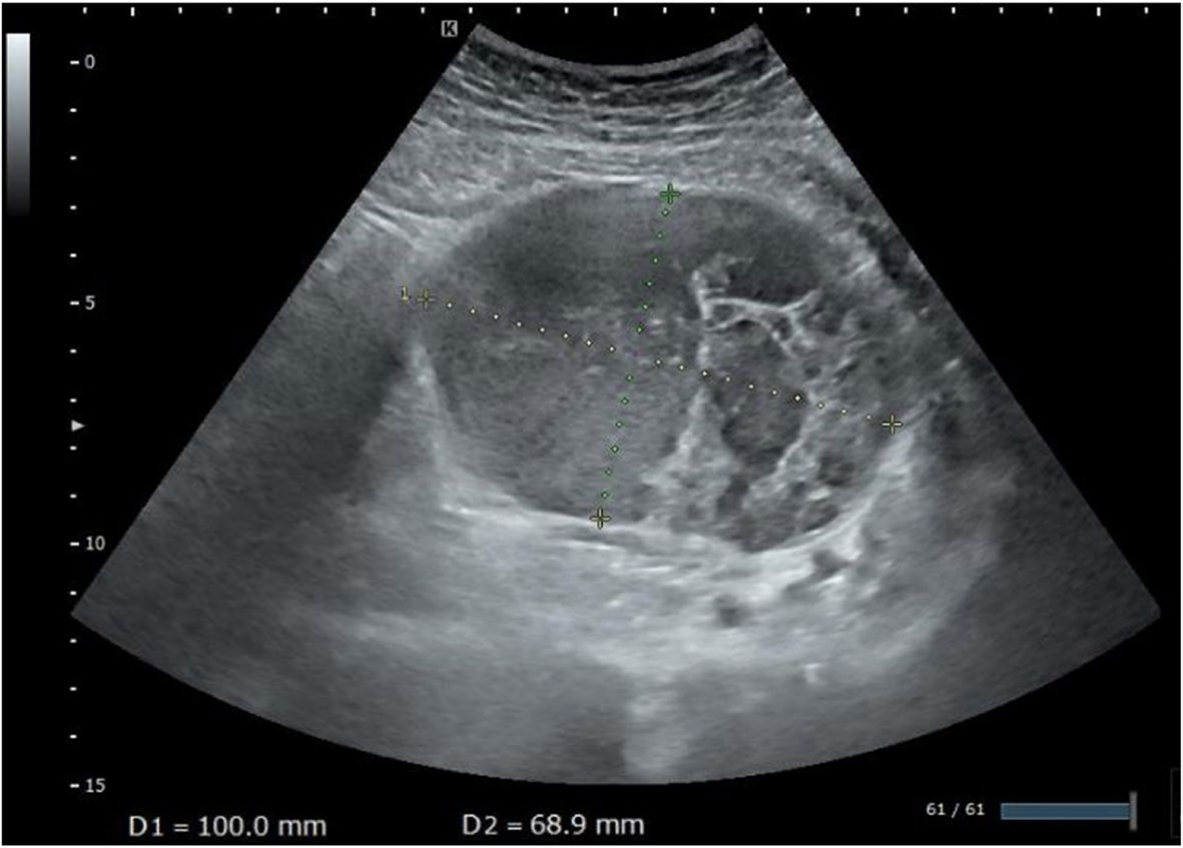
Transabdominal ultrasonography findings. A large mass in the left lower abdomen predominantly consisted of multiple cystic lesions of various sizes filled with echogenic liquid, a cavernous compartment and multiple septations and measured 10.0 × 6.9 cm
